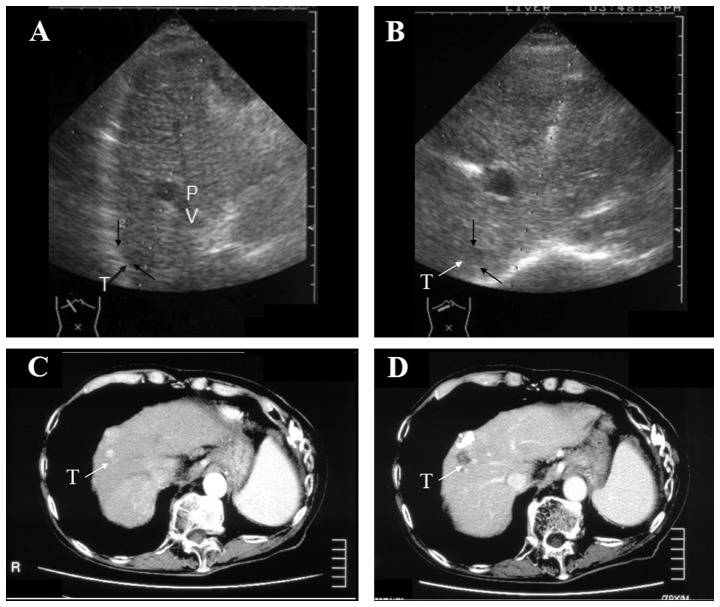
Image findings for patient 1. (A) Ultrasonograms captured by right intercostal scanning prior to therapy. A large portal vein (PV) intersects the puncture point and the nodule (arrow). A curved percutaneous ethanol injection therapy (PEIT) needle was used to conduct the right subcostal scanning during therapy. (B) The needle tract of an overcoat needle is observed at the left side of the right PV. (C) A small contrast enhanced tumor (1.6×1.6 cm) is located in S4 in the arterial phase of an abdominal, dynamic computed tomography image in patient 1 prior to therapy I (arrow). (D) A white colored nodule on the subcapsular region of the liver demonstrates the remaining iodized oil (Lipiodol) that accumulated during the previous PEIT (with a mixture of ethanol and Lipiodol) in 2005. Subsequent to the therapy, the tumor evolved into a low-density area without contrast enhancement (arrow).
